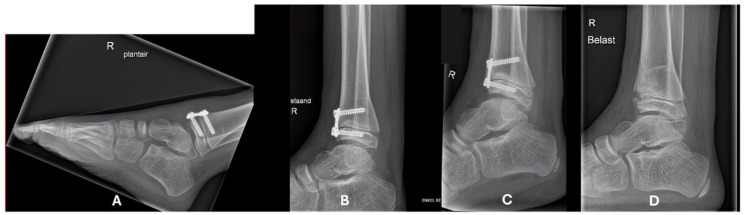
Guided growth for equinus deformities. Radiographs are directly postoperative (a), after 14 months of follow-up in standing position (b), after 23 months of follow-up in standing position (c), and at 40 months of follow-up after removal of TBP in standing position (d). A gradual increase in the anterior distal tibial angle can be observed, with a corresponding clinical improvement in ankle dorsiflexion.
